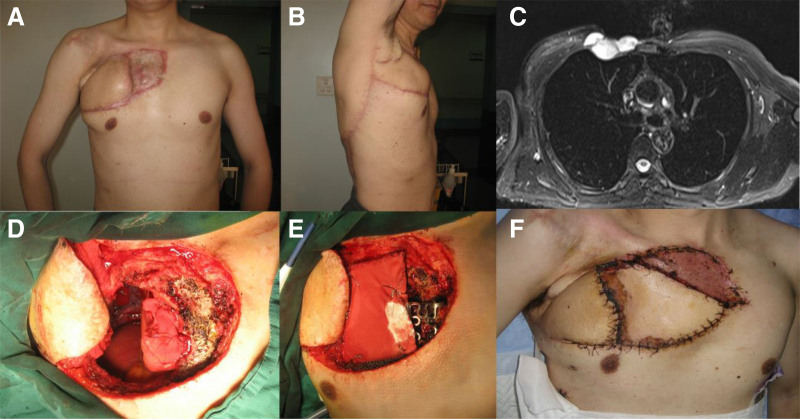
Clinical presentation and MRI findings of case 2. (A–B) DFSP lesion located in the chest region; (C) Magnetic resonance imaging before surgery; (D) 2nd and 3rd ribs and adjacent pleura were resected during surgery; (E) Chest wall defect was covered by titanium and polyester meshes; (F) Immediate post-operative picture with the flap sutured to the chest wall defect.
